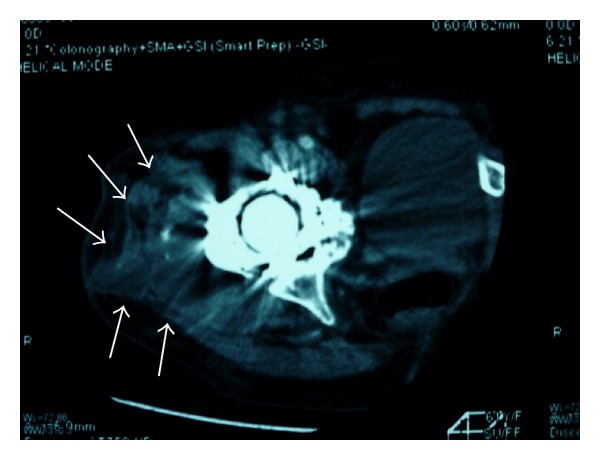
We tested for an arterial bleed with computed tomography using a contrast medium. Although active bleeding was not observed, a hematoma around the hip joint was visible in this test (white arrows).
